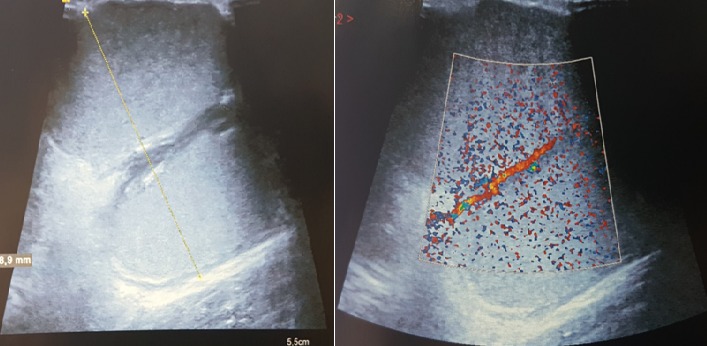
Échographie du coude: aspect bilobé, hétérogène de la masse, bien vascularisée au doppler
